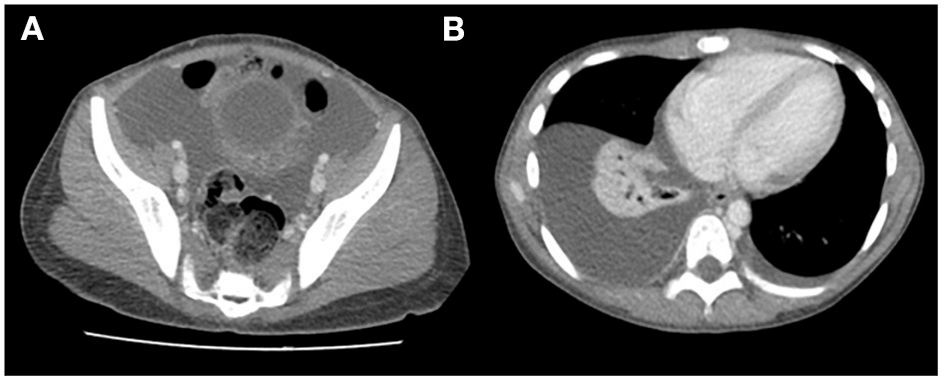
Abdominal CT: ascites (A), bilateral pleural effusion (B), and lumbar subcutaneous edema (A).
